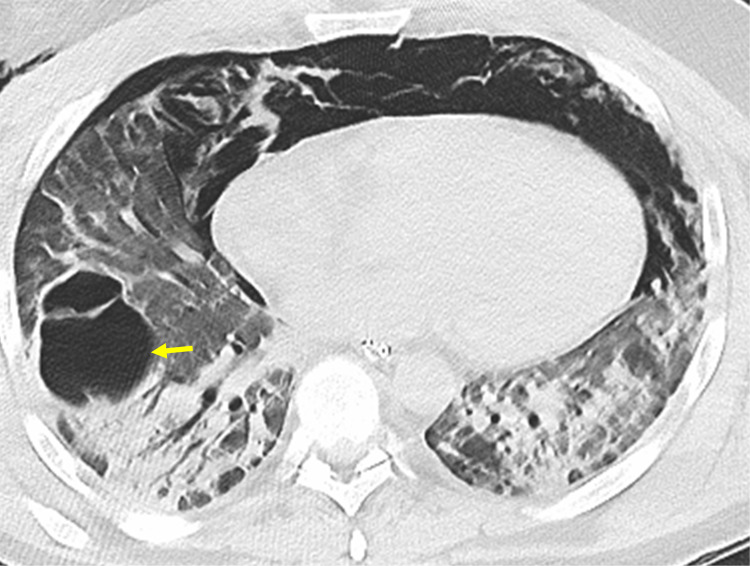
CT scan of the chest without contrast CT scan of the chest without contrast (axial image) shows extensive pneumomediastinum and small right pneumothorax. Pneumatocele (yellow arrow), mild bronchiectasis, ground-glass opacities, and consolidations in bilateral lower lobes can be seen.
